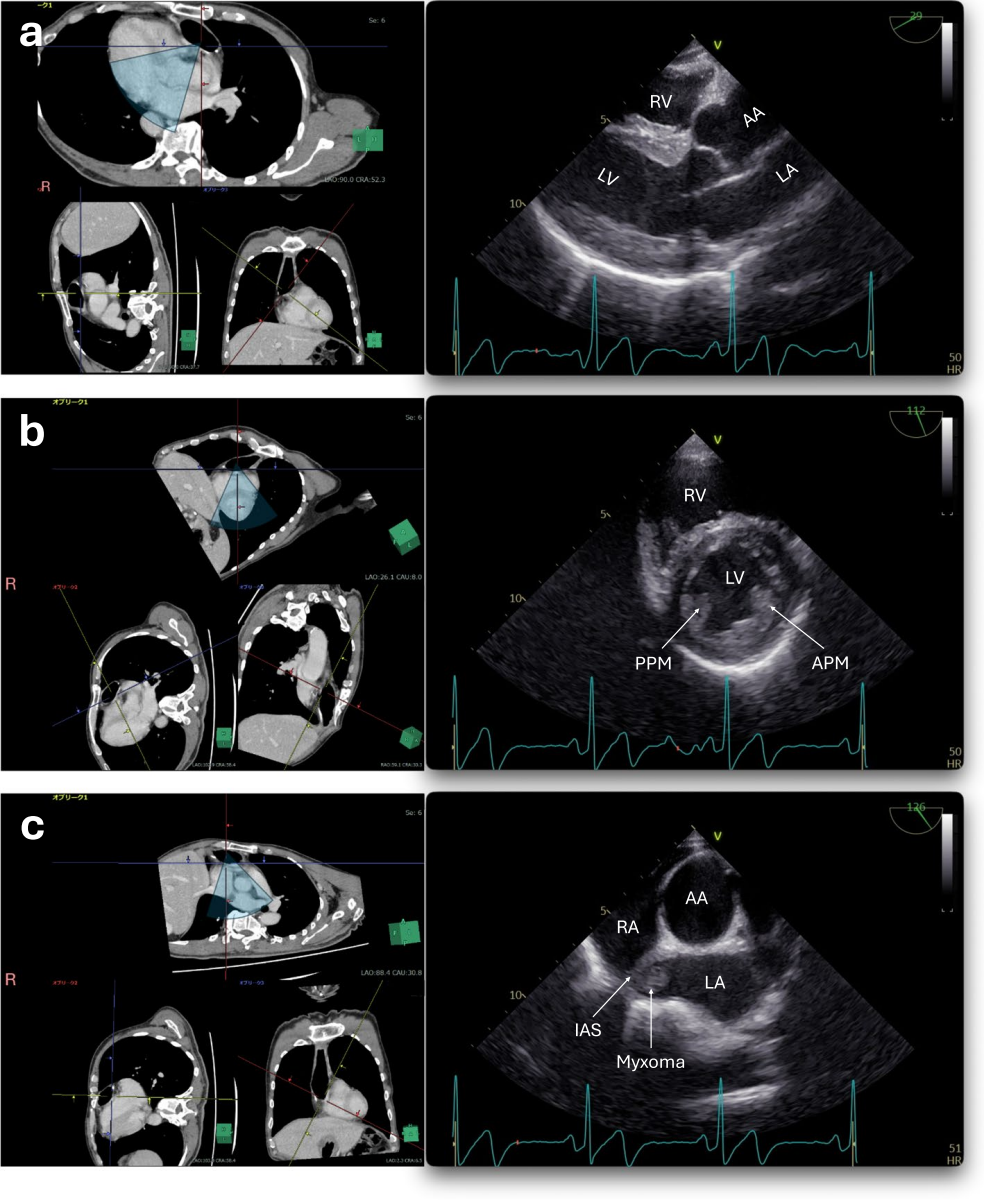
Transesophageal echocardiographic images, similar to left parasternal views, and corresponding sections on computed tomography. The right pane of each subfigure shows transesophageal echocardiographic images, while left pane shows corresponding sections on preoperative computed tomography (CT). a Long-axis view of the left ventricle, b Short-axis view of the left ventricle, c Short-axis view of the ascending aorta with a tumor seen in the left atrium. The inclinations of the yellow lines in the bottom-left and bottom-right CT images indicate the probe rotation and transducer array angles, respectively, when obtaining ultrasound images corresponding to the top CT images. The blue-shaded fan shapes in the top CT images replicate the ultrasound scanning area. AA, ascending aorta; APM, anterolateral papillary muscle; IAS, interatrial septum; LA, left atrium; LV, left ventricle; PPM, posteromedial papillary muscle; RA, right atrium; RV, right ventricle
